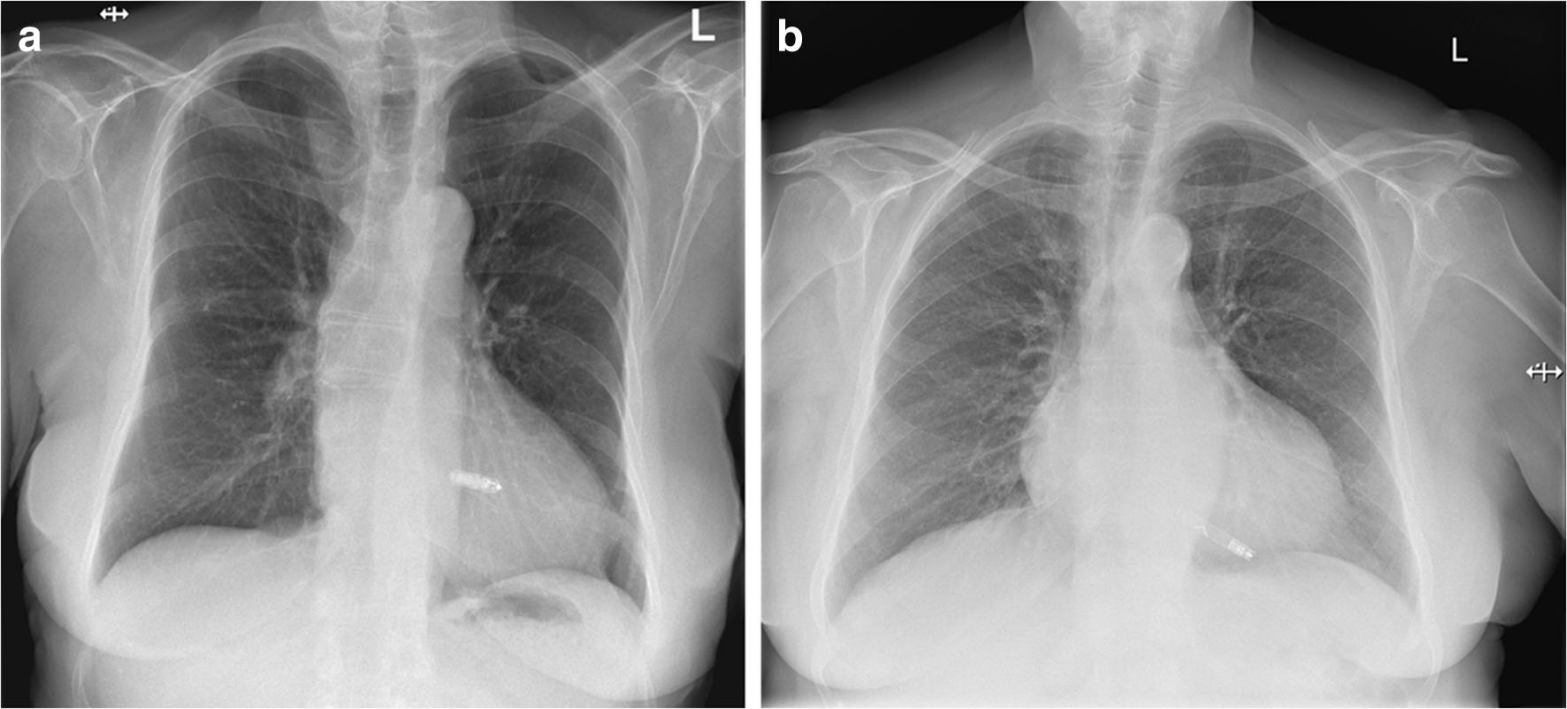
X-ray pictures of an implanted Micra^®^ TPS LP (a) and a Nanostim^®^ LCP system (b) are shown. The authors thank Prof. Dr. W. Jung, Schwarzwald-Baar Klinikum, Villingen-Schwenningen, Germany, for providing material for Fig. 2b
